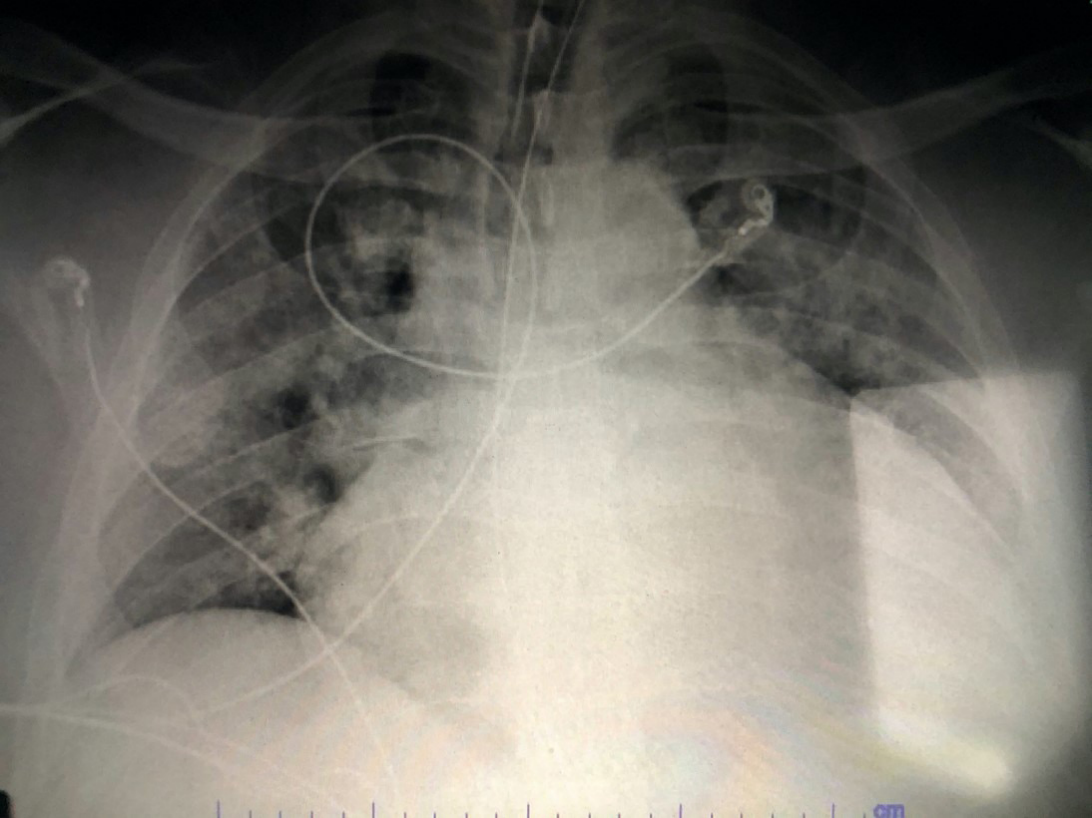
Anterior-posterior chest radiograph of a 48-year-old male with respiratory distress and altered mental status.
